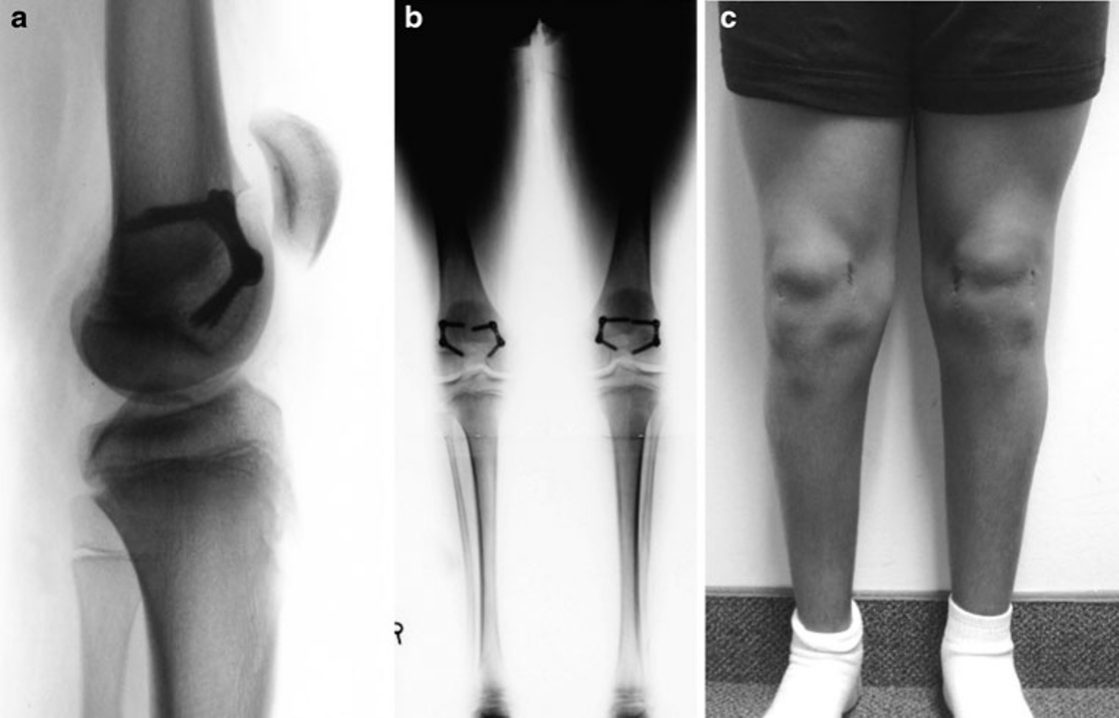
a Lateral view documenting full correction of the fixed knee flexion deformity. Despite the residual patella alta, he has no pain, patellar instability, or related functional limitations; therefore, the patellar tendon does not need to be advanced. b A full-length radiograph showing the retained 8-plates. c Clinical appearance at the time of follow-up gait analysis. Upon full correction of the fixed knee flexion deformity, the 8-plates are typically removed. All images are from subject 1
